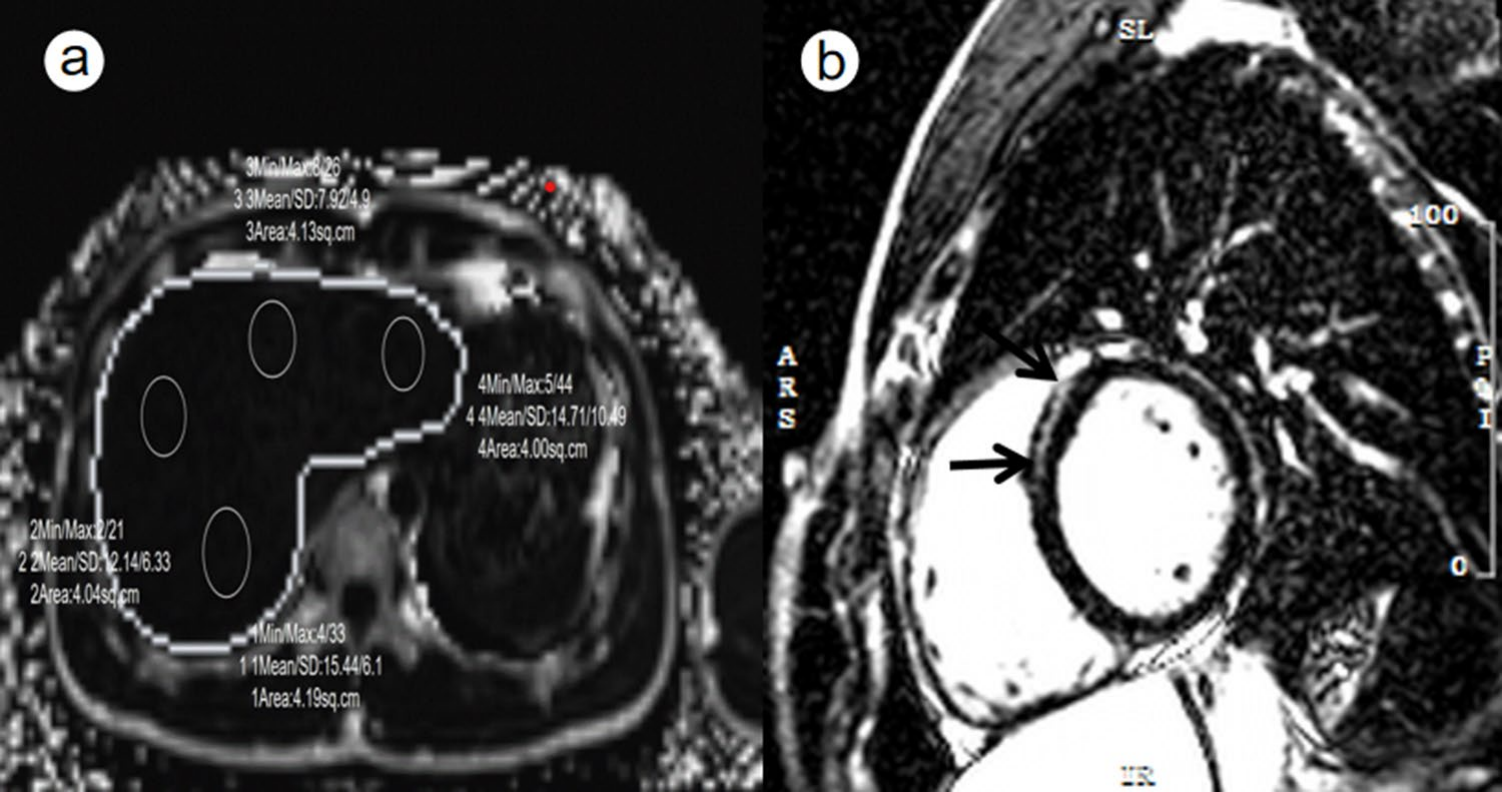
MRI images in a 26-year-old male athletes.The average liver fat content in MRI-PDFF image is 1.25% (the measured values of the ROIs 1–4 were 1.5%, 1.2%,0.8% and 1.5%, respectively)(Figure a, circle). Short axis images of late gadolinium enhancement depict a striped high signal in the middle myocardium of septal wall for left ventricle (Figure b, arrow)
